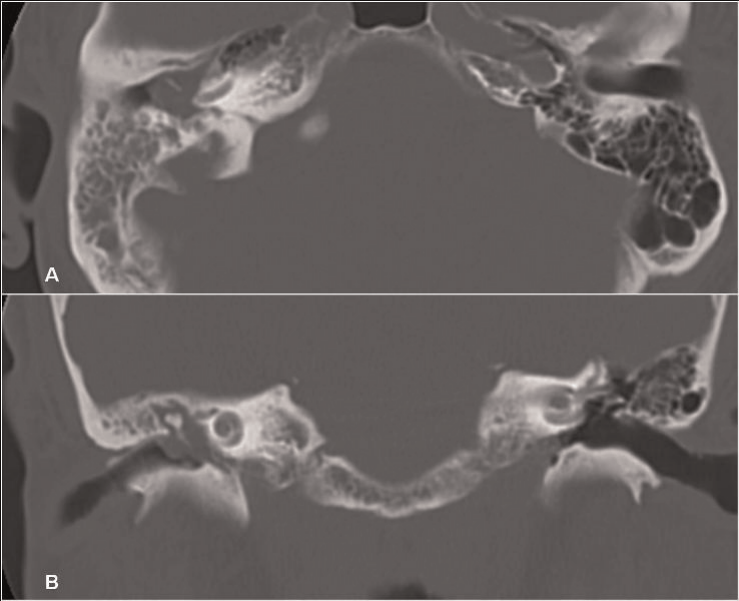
Axial (A) and coronal (B) views of a mastoid computerized tomography (CT) scan showing a right mastoid and middle ear opacity. The tympanic membrane is pressed laterally by the mass.
